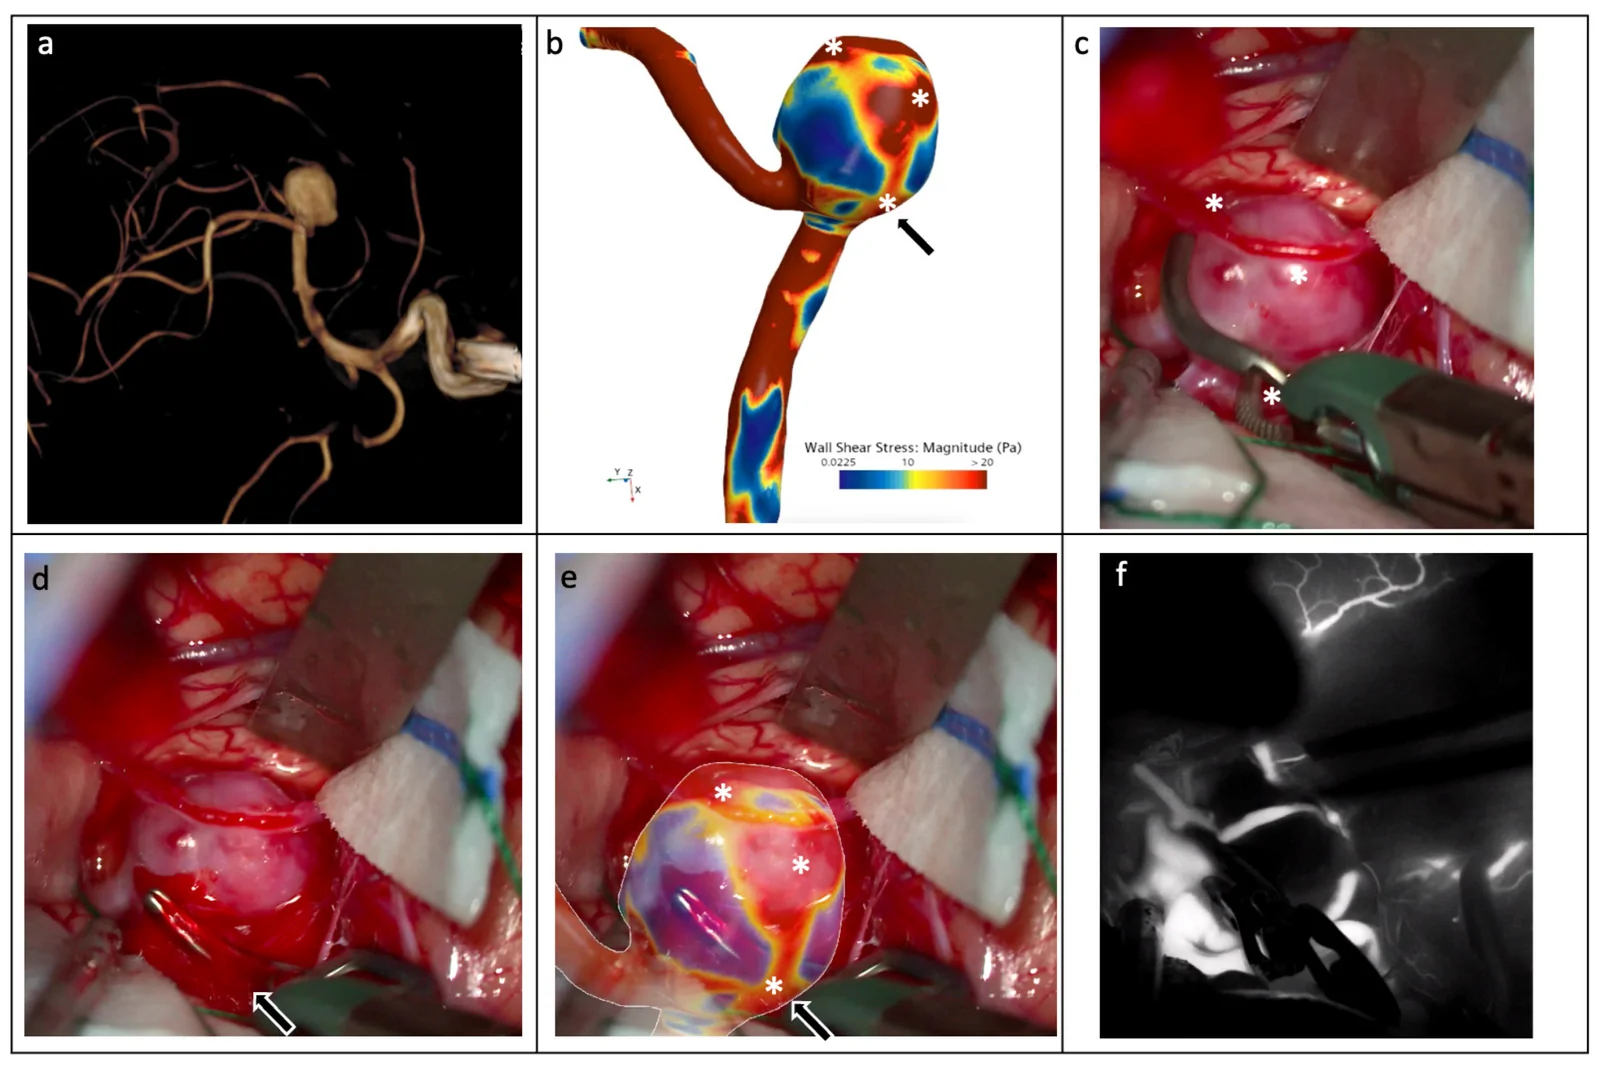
(a) Preoperative brain CTA showing right MCA UIA, which was further adopted for segmentation and CFD modeling. (b) UIA CFD model showing heterogeneous wall shear stress with focal areas of high wall pressure. (c) intraoperative UIA dissection, showing marked fragility of UIA dome. (d,e) intraoperative spontaneous rupture at the base of the dome, at the point of elevated wall pressure, as demonstrated by the overlay of former 3D CFD model. (f) successful clipping with complete exclusion of MCA aneurysm. *: areas of elevated shear stress and high wall pressure; black arrow: point of intraoperative spontaneous rupture.
